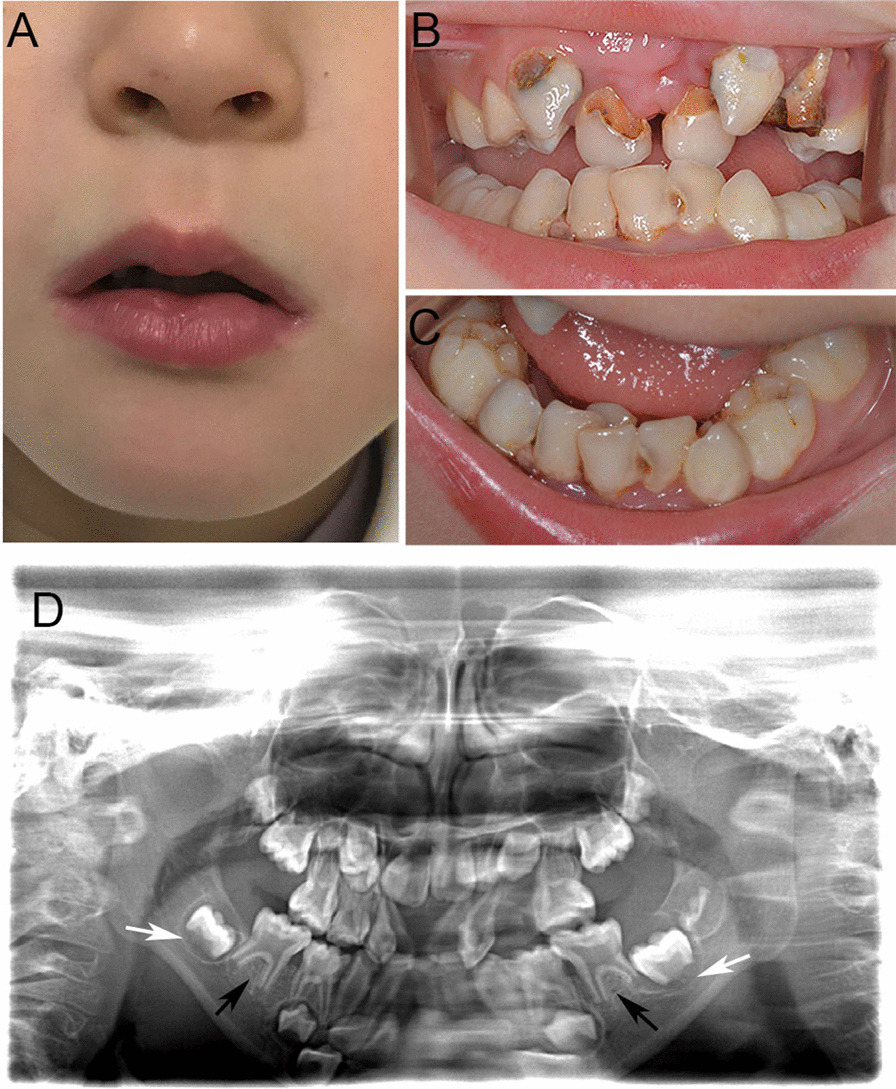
Clinical and radiographic features of case 2 with SAS. A The extraoral photo showing cherry-like lips and thin upper lip; B–C The intraoral photo showing extensive caries, crowed teeth, malposed teeth and poor oral hygiene; D Panoramic radiographs showing loss of mandibular second bicuspids, delayed root formation of primary teeth 75 and 85 (black arrows), and permanent teeth 36 and 46 (white arrows)
